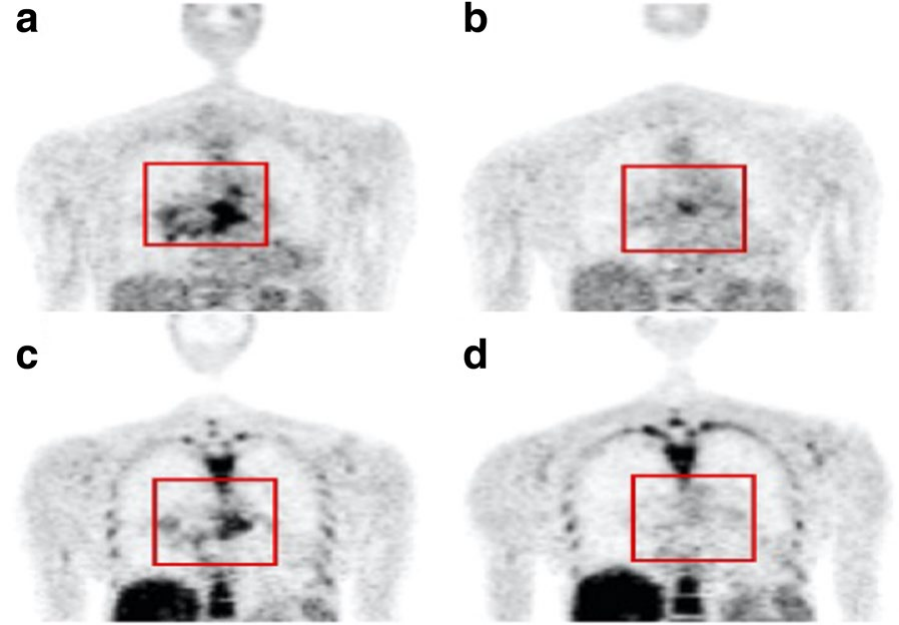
Evaluating response to erlotinib therapy with [^18^F]FDG-PET and [^18^F]FLT-PET. [^18^F]FDG-PET (a) and [^18^F]FLT-PET (c) before start of treatment, and [^18^F]FDG-PET (b) and [^18^F]FLT-PET (d) after 1 week of treatment with erlotinib. [^18^F]FDG uptake is higher than [^18^F]FLT in the baseline scans. Erlotinib treatment decreases uptake of both radiotracers. The [^18^F]FLT scan after therapy shows minimal uptake indicating that erlotinib is effectively inhibiting proliferation. Reprinted with permission from JNM. This research was originally published in JNM. Kahraman et al. [43]
